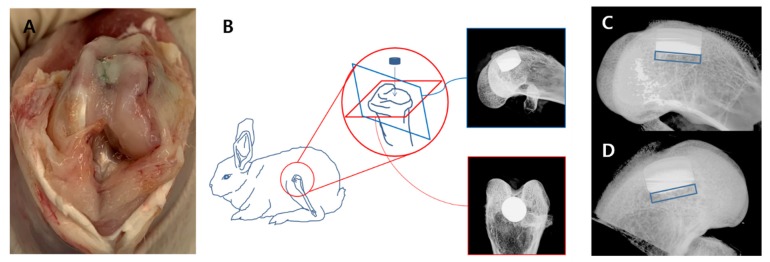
A: After euthanizing, the right side of the distal femur was harvested. B: Axial and lateral images were obtained using micro X-ray. C, D: Osteolysis was defined as a low-density area visible within a 1-mm area from bone-implant contact surface (lateral-view).
